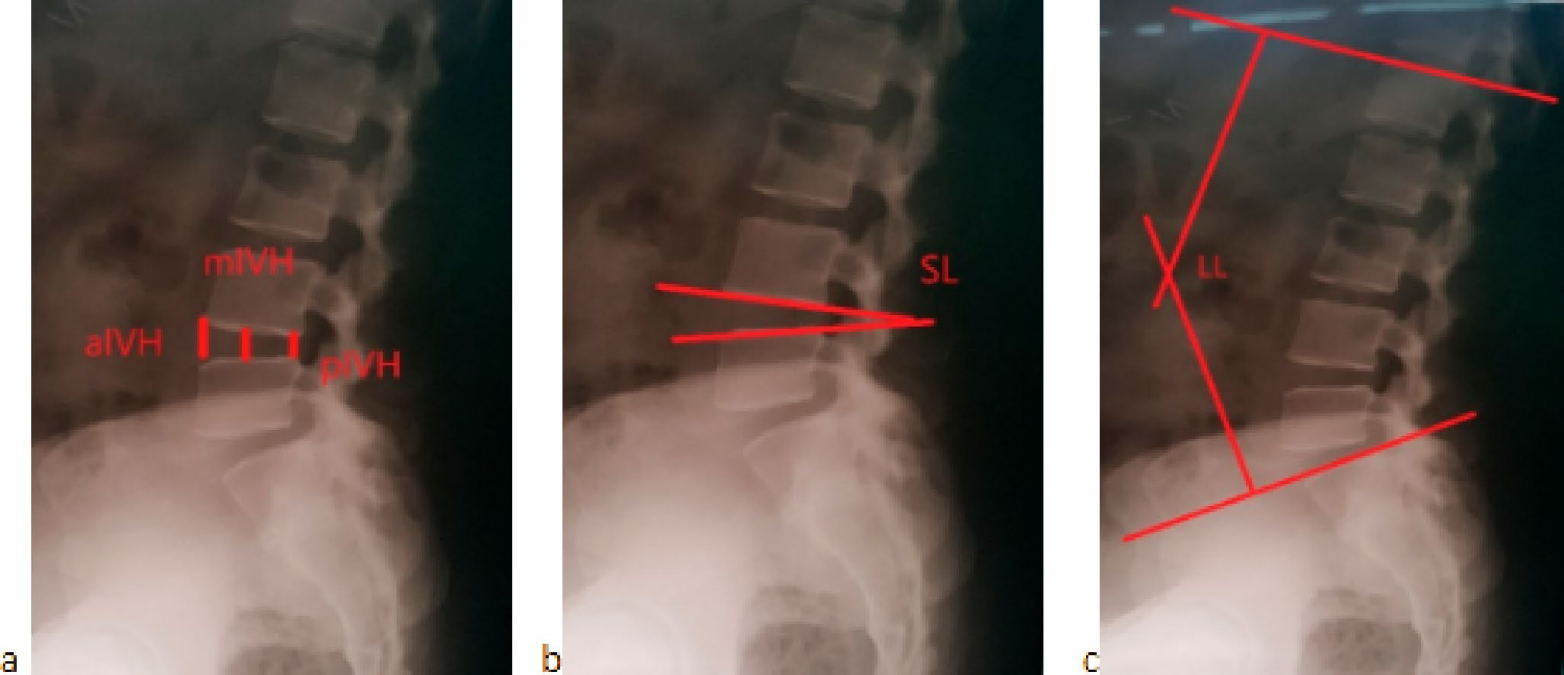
Plain X-rays on the lumbosacral spine lateral views showing a-measurement methods of the pathological segment’s anterior, posterior, and midpoint intervertebral height (aIVH, pIVH, and mIVH), b- measurement of segmental lordosis (SL) c- measurement of lumber lordosis (LL)
